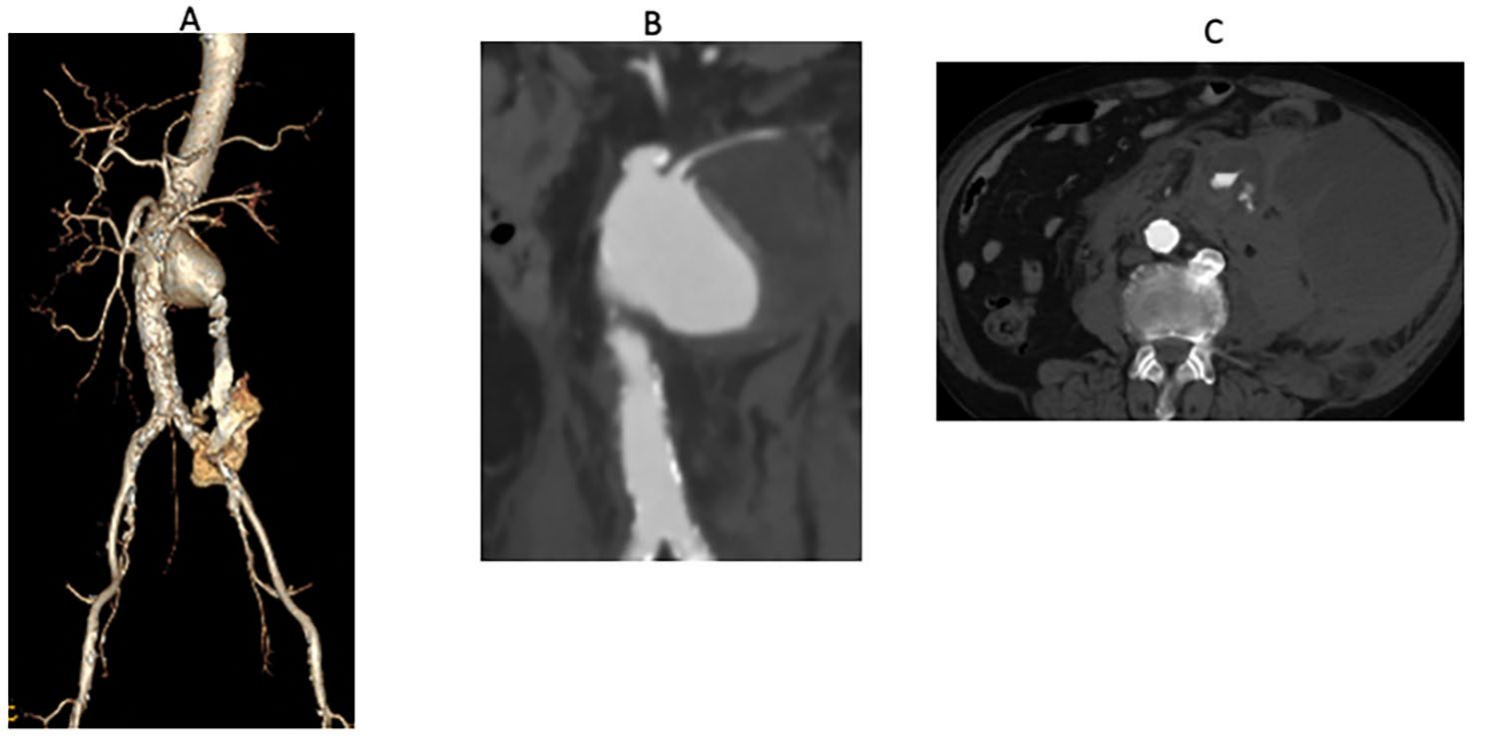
Preoperative CT. A and B: Preoperative computed tomography (CT) shows a ruptured thoracoabdominal aortic aneurysm (rTAAA) and the left renal artery arising from the thoracoabdominal aortic aneurysm (TAAA). C: The patient had a ruptured thoracoabdominal aortic aneurysm with a massive retroperitoneal hematoma
